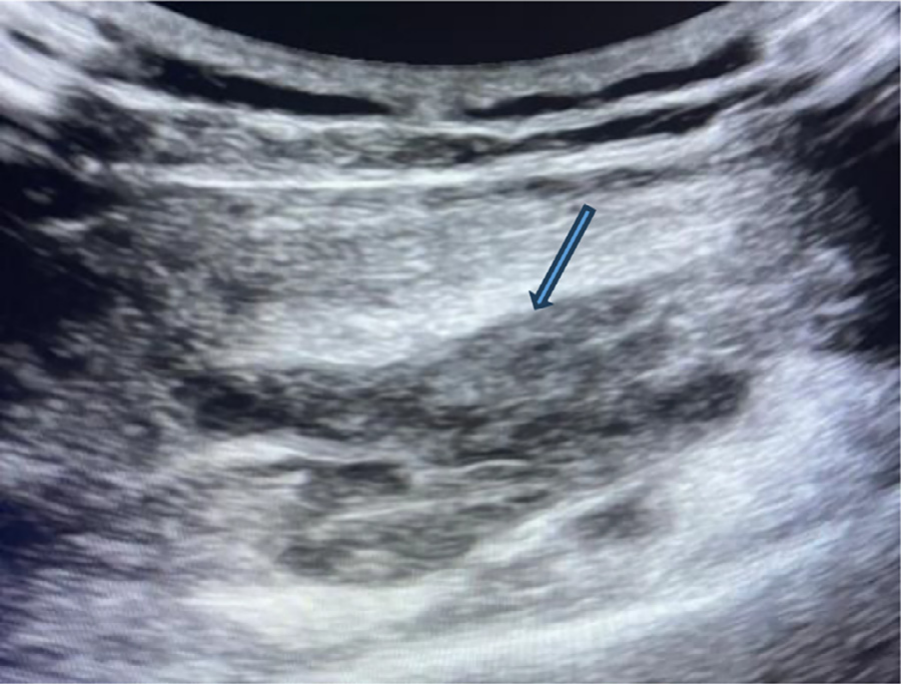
Ultrasound image depicting an oval, macro-lobulated, hypoechoic intramuscular mass with echogenic trabeculae.
